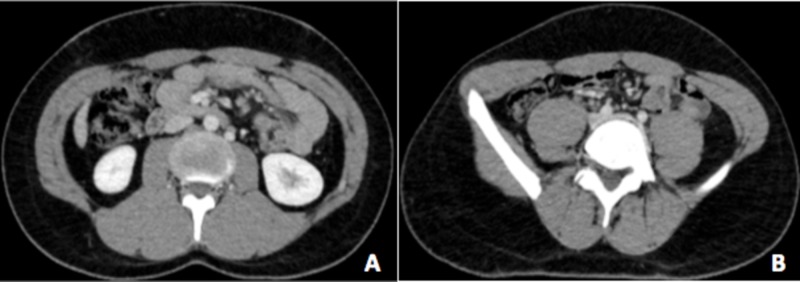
Follow-up computed tomography (CT) scan of the abdomen six months post-discharge showing significant regression of abdominal lymphadenopathy
